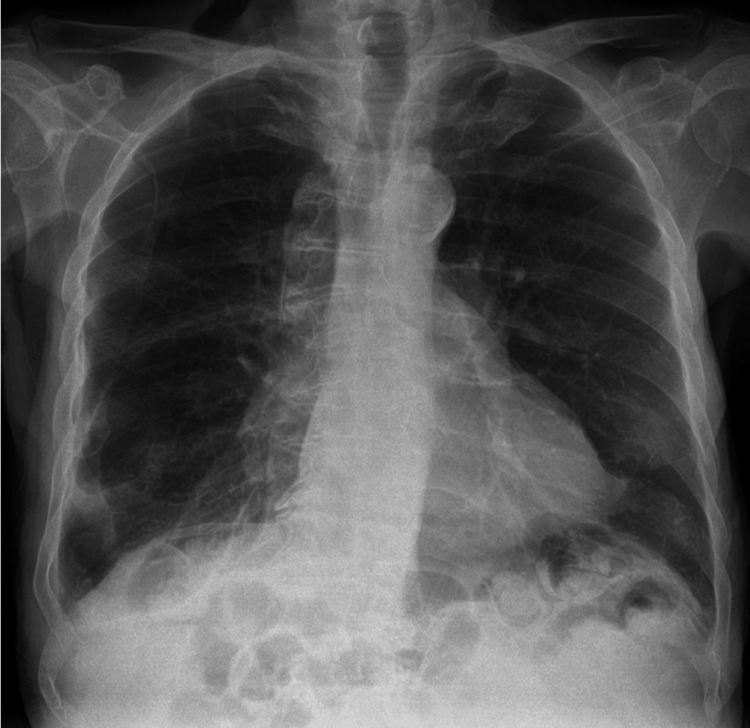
Posteroanterior chest X-ray not showing skinfold lining or pneumothorax.
